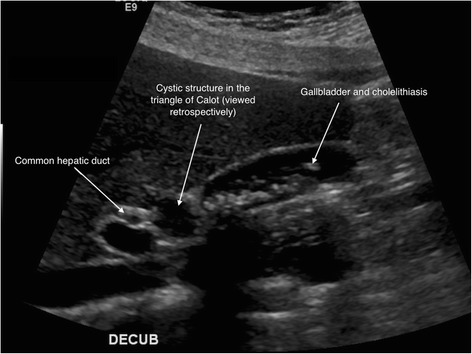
An image of the pre-operative ultrasonographic scan of the liver and biliary ducts showing multiple gallstones, normal biliary structures and portal vein and a cystic structure that could be the ciliated foregut cyst (noticed retrospectively)
